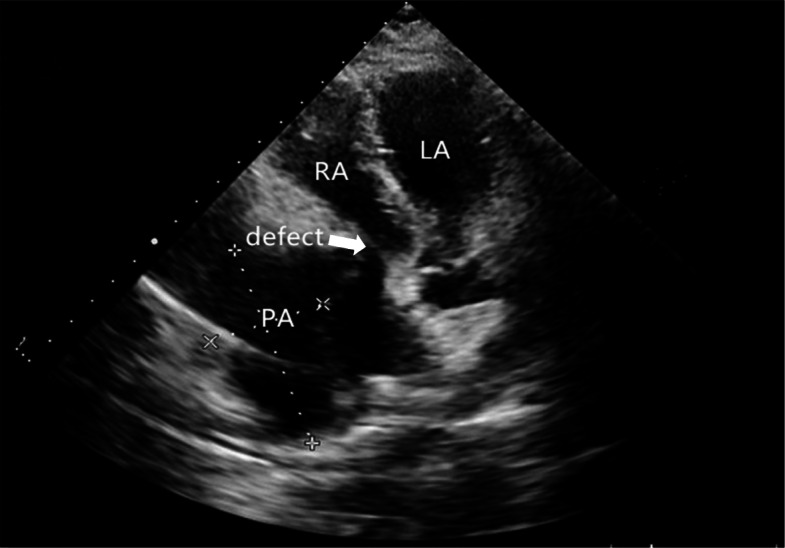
Three-dimensional transthoracic echocardiogram. TTE shows an irregular cystic mass with a pseudoaneurysm measuring 8.49*3.81 cm in size in the lateral wall of the right atrium, and the mass obviously compressed the right atrium. A defect was observed between the pseudoaneurysm and the right atrium. LA: left atrium; RA: right atrium; PA: pseudoaneurysm
